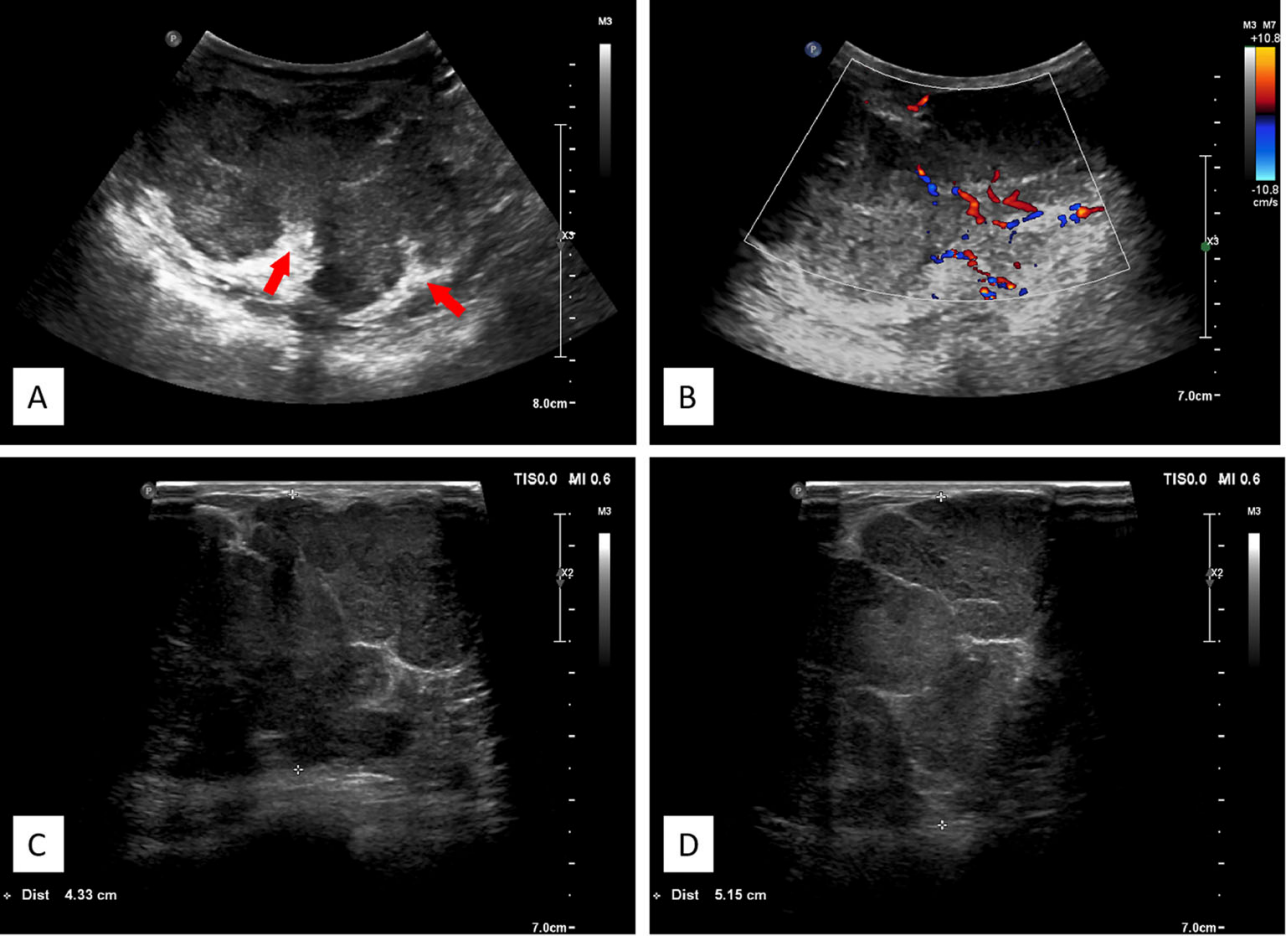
(A) Ultrasonographic image showing multiple hypoechoic lesions in the right breast. The large masses comprising nearly the entire right breast, with lobulated irregular margin, the banded median-high echogenic septum was seen inside the mass. (B) Color flow Doppler image showing abundant blood flow signals. (C, D) Different spliced images of the tumor showed irregular margin with multiple septa.
